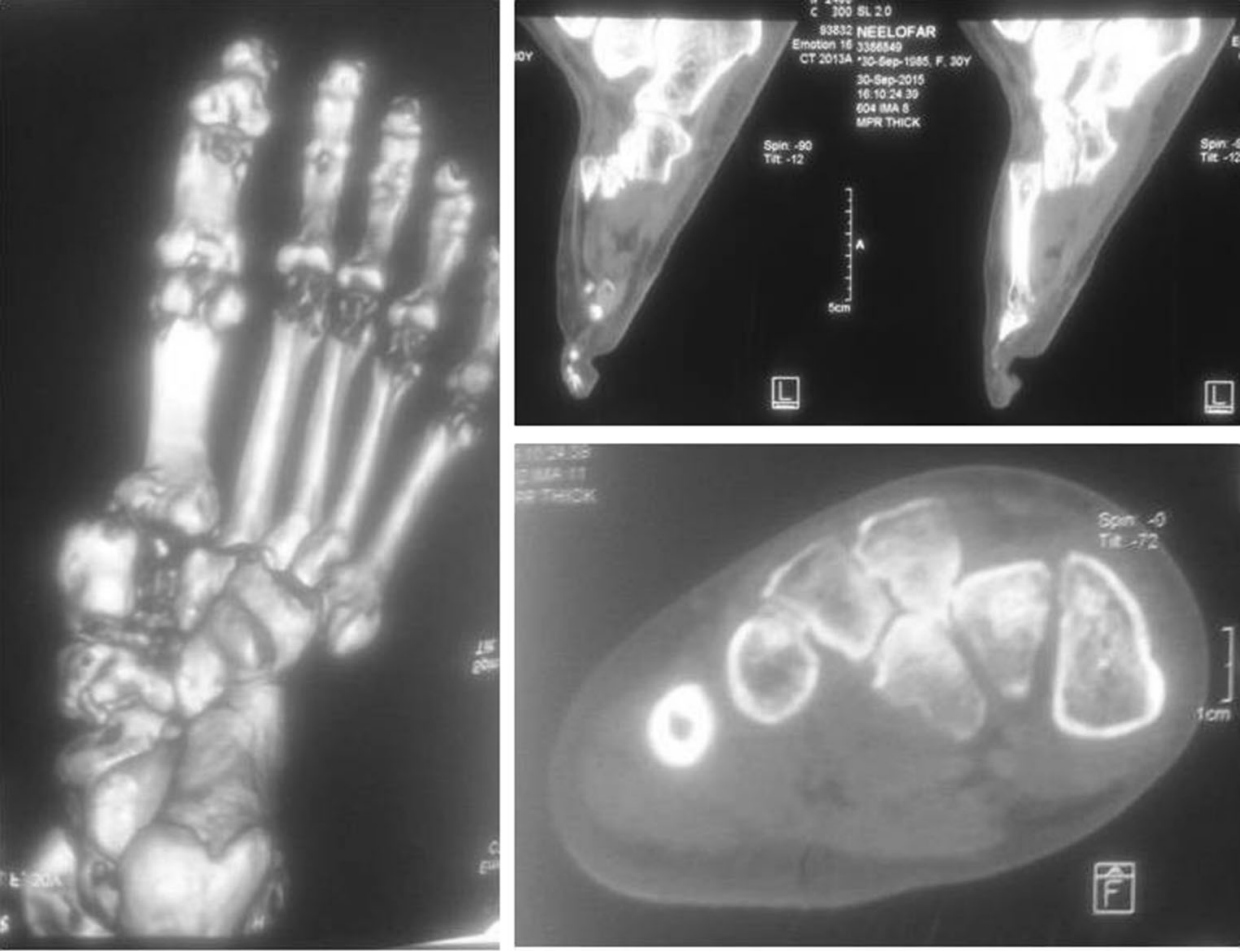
CT images of involved foot on presentation
